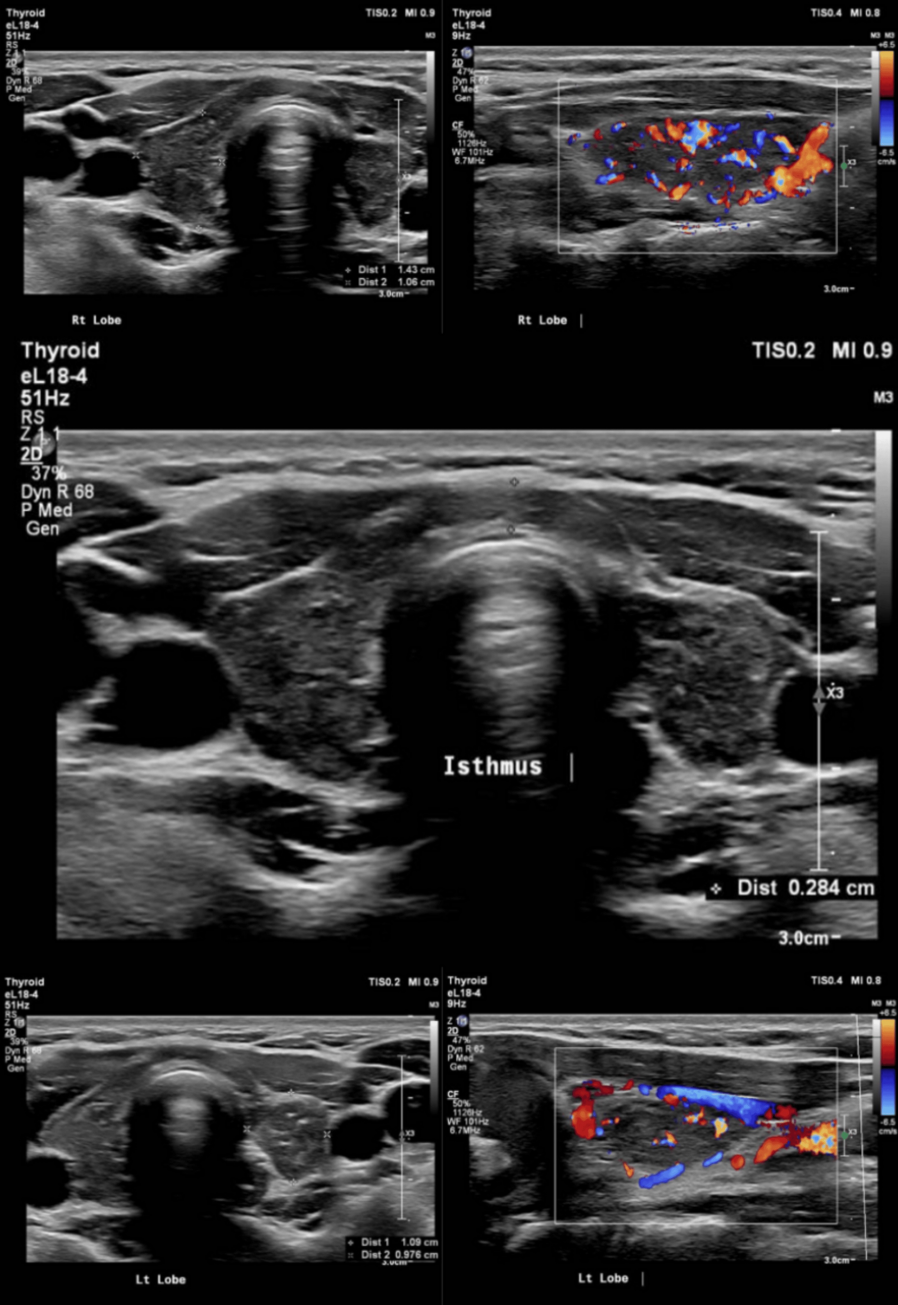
Ultrasound of the thyroid showing lobes and isthmus appearing normal in size having heterogeneous echotexture. Diffuse increased vascularity is also seen, suggestive of thyroiditis
